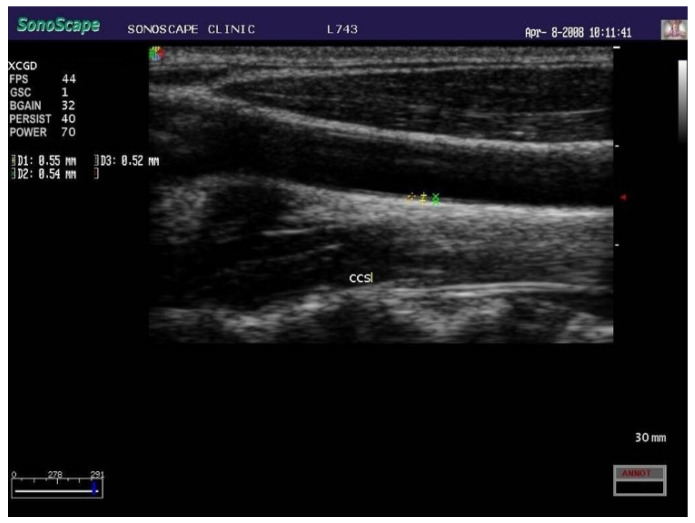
Carotid increased intima-media thickness in a child with FHC (ultrasound scan).
